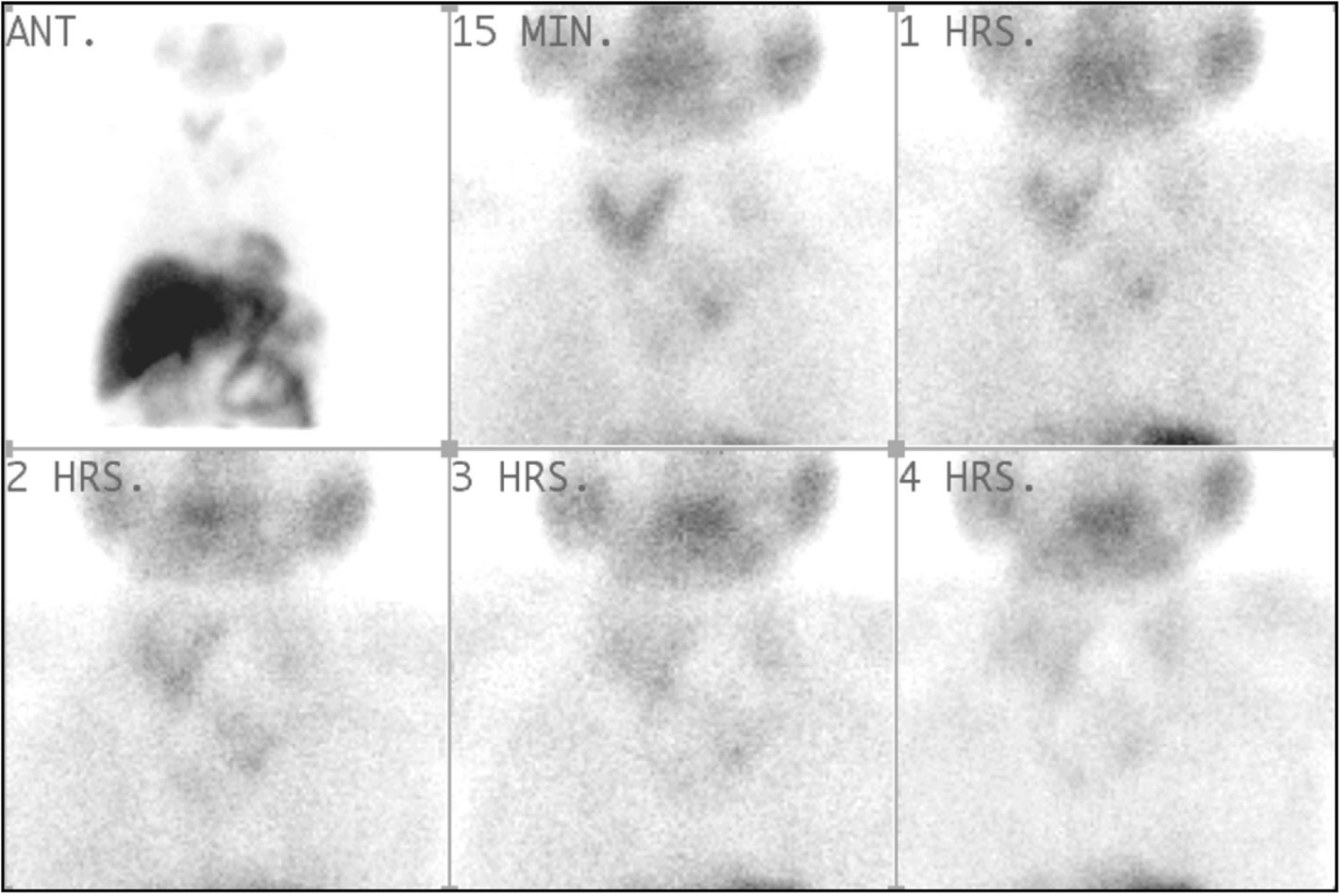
Radionuclide parathyroid scintigraphy showing cystic lesion over left side of neck.
